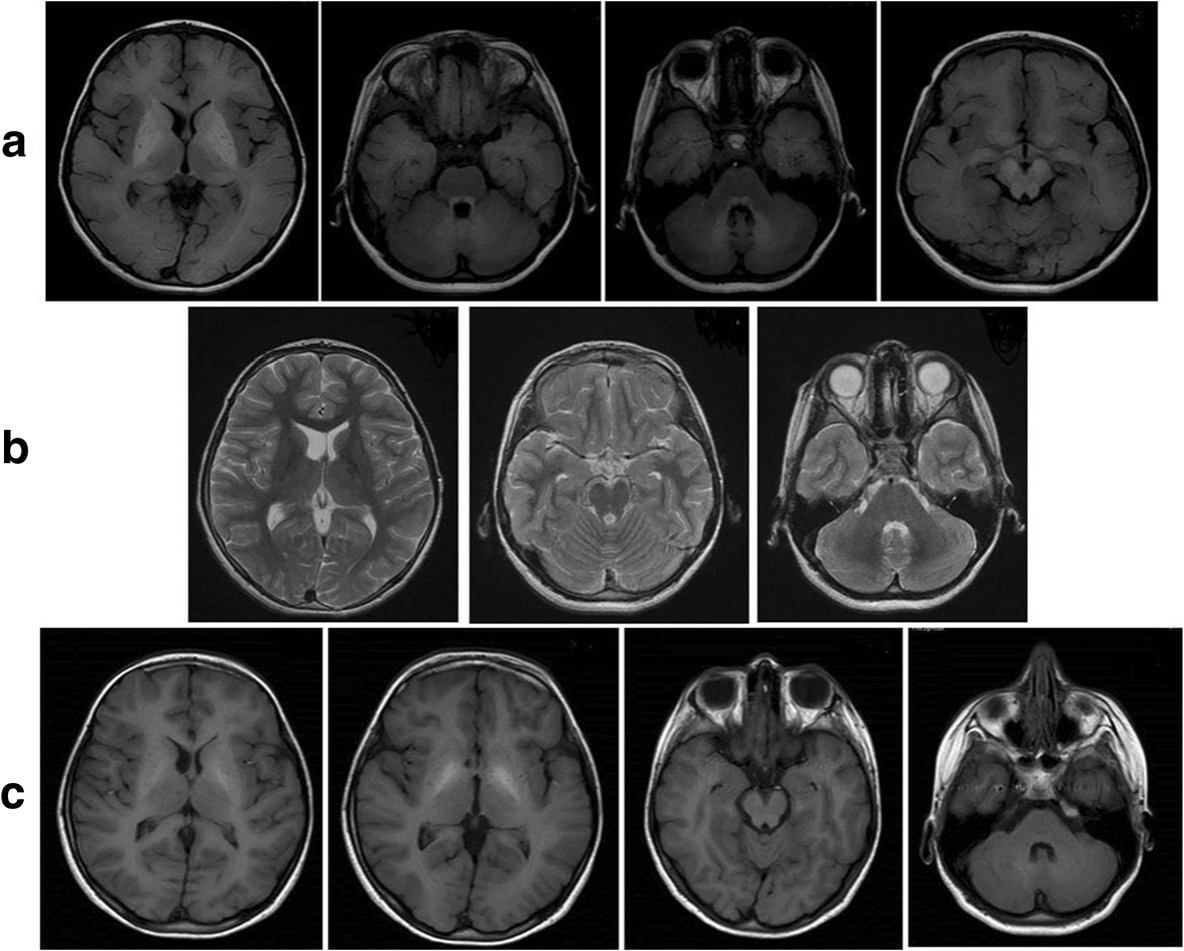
Brain MRI of the patient showed symmetric hyperintensities of the basal ganglia, tectum of the pons, and dentate nucleuses on T1- W images (a) and a normal appearance on T2-W images (b). Repeated brain MRI at 6 months follow-up showed significant resolution of previous findings on T1 images (c)
